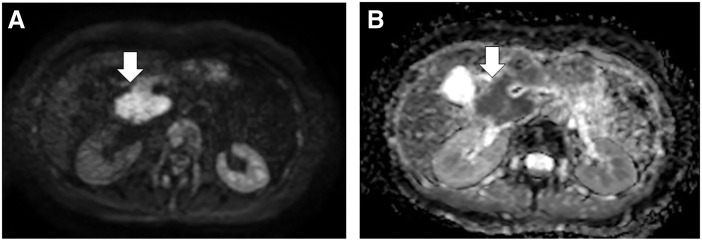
On MRI, (A) DWI shows a high-signal mass measuring 48 × 28 mm in the pancreatic head (arrow), and (B) the ADC map shows a low-signal mass with clear margins (arrow). ADC, apparent diffusion coefficient; DWI, diffusion-weighted imaging
